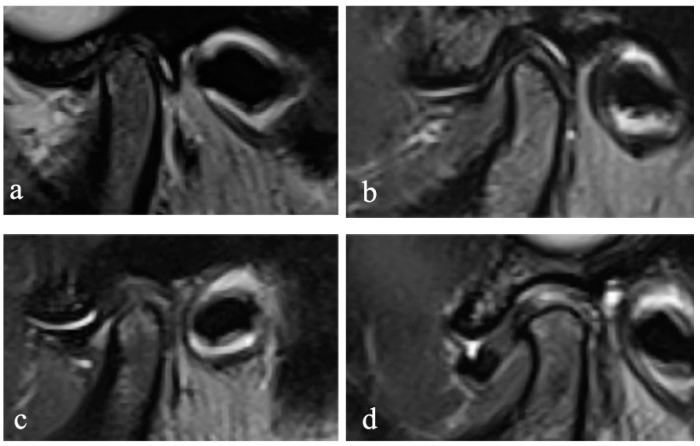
Joint effusion. Sagittal T2-weighted image MRI showing: (a) no fluid signal, (b) dots or lines of bright signal, (c) bands of bright signal, and (d) pooling of bright signal on the TMJ.
